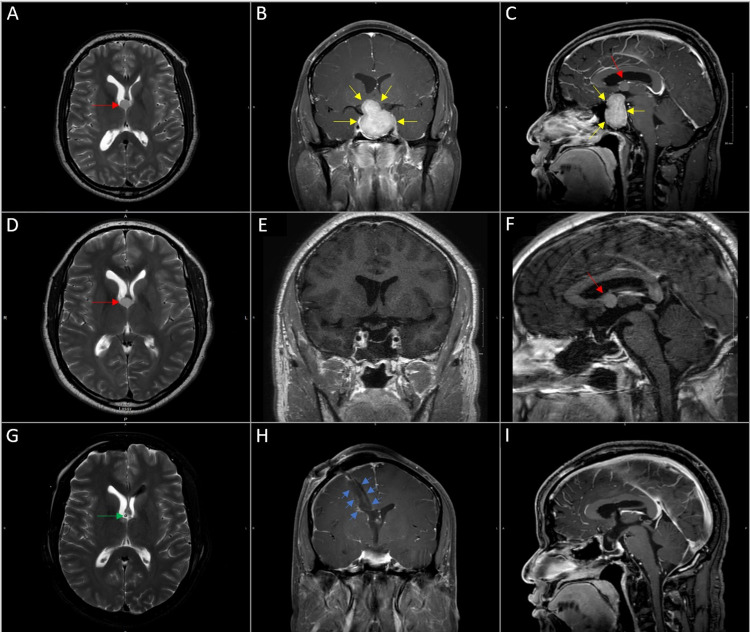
Coexistent colloid cyst and pituitary macroadenoma. MRI of the brain with and without contrast at presentation demonstrates the colloid cyst (red arrows) on an axial T2 sequence with slight enlargement of the frontal horn of the right lateral ventricle but without overt evidence of acute hydrocephalus such as transependymal edema (A), the pituitary macroadenoma (yellow arrows) on a coronal post-contrast T1 sequence (B), and coexistent lesions on a sagittal post-contrast T1 sequence (C). An MRI of the sella with and without contrast after resection of the pituitary macroadenoma demonstrates the stable appearance of the colloid cyst on an axial T2 sequence and sagittal post-contrast T1 sequence (D,F) with evidence of residual macroadenoma around the cavernous sinus bilaterally (E,F). The optic apparatus appeared well-decompressed after EETS of the pituitary macroadenoma (E). An MRI of the brain with and without contrast after subsequent resection of the colloid cyst utilizing the BrainPath® tubular retractor system demonstrates gross total resection with the placement of a temporary ventriculostomy catheter (tip of catheter denoted by the green arrow) in an axial T2 sequence (G) and a coronal post-contrast T1 sequence (H). A sagittal post-contrast T1 image demonstrating both areas of resection simultaneously (I). A small transcortical tract (blue arrows) on a trajectory towards the foramen of Monro demonstrates the minimally invasive access to the right lateral ventricle utilizing a tubular retractor (H).
